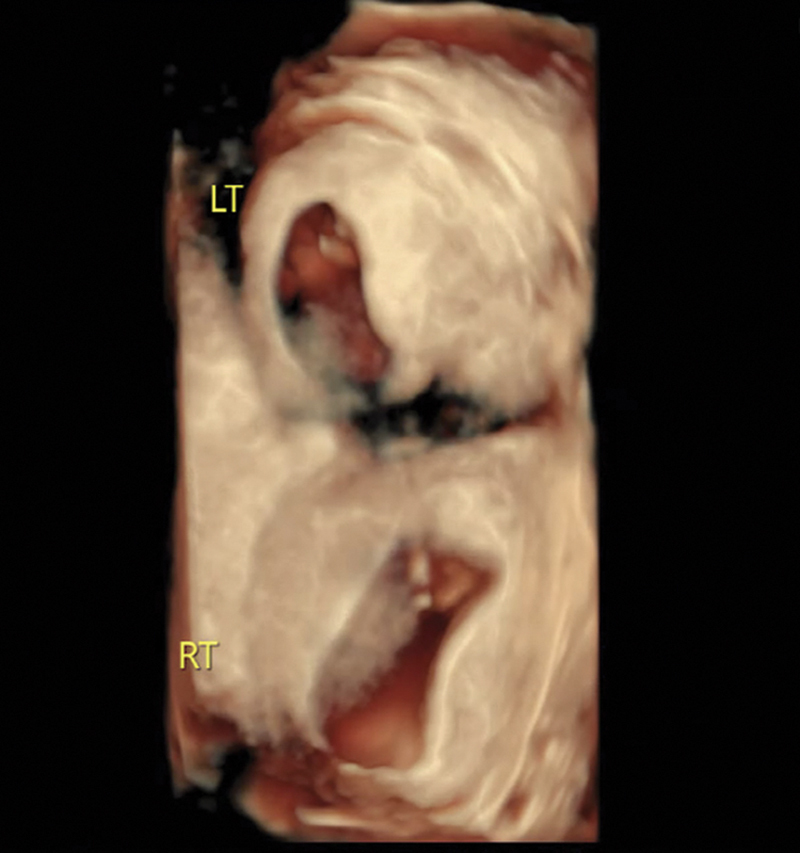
Three-dimensional rendering ultrasound at 11
